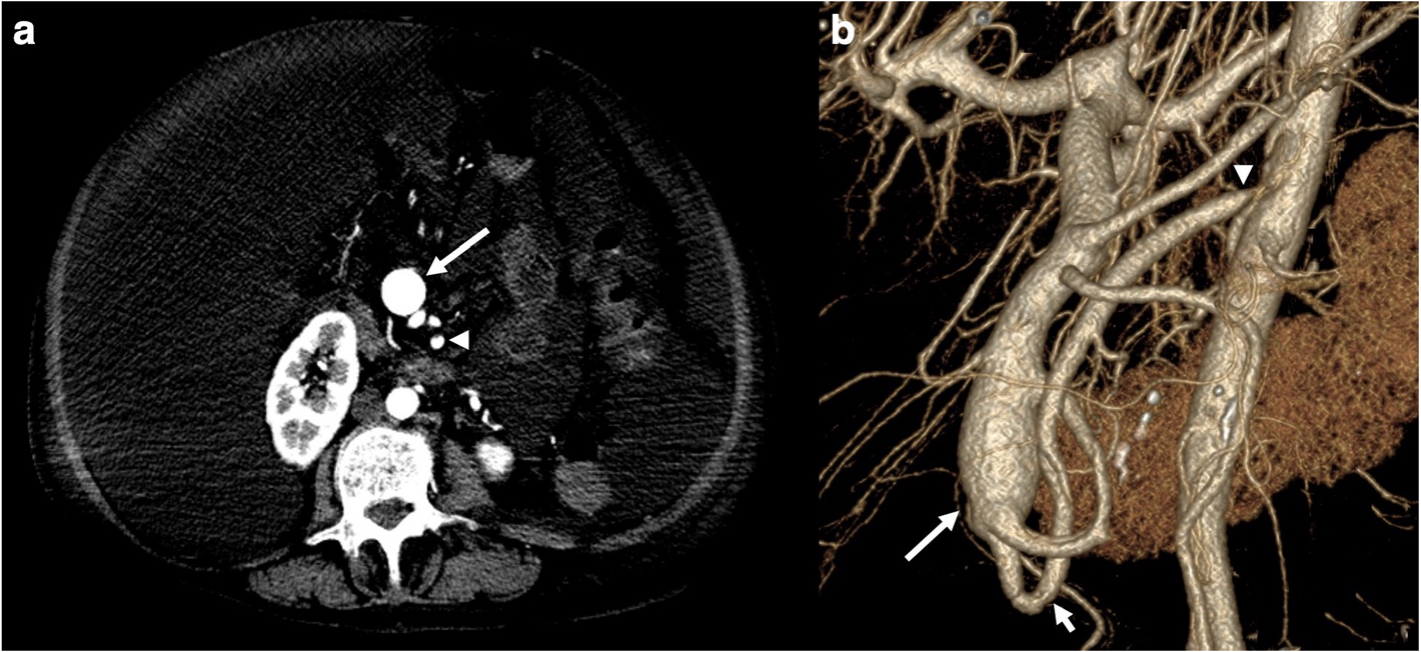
Contrast-enhanced abdominal CT scan (arterial phase) of a superior mesenteric arteriovenous fistula (SMAVF) (a) Axial image shows a SMAVF (arrowhead) feeding into a dilated superior mesenteric vein (arrow) (b) 3D reconstruction shows the SMAVF (short arrow) connecting the superior mesenteric artery (arrowhead) to an ectasia of the superior mesenteric vein (long arrow)
